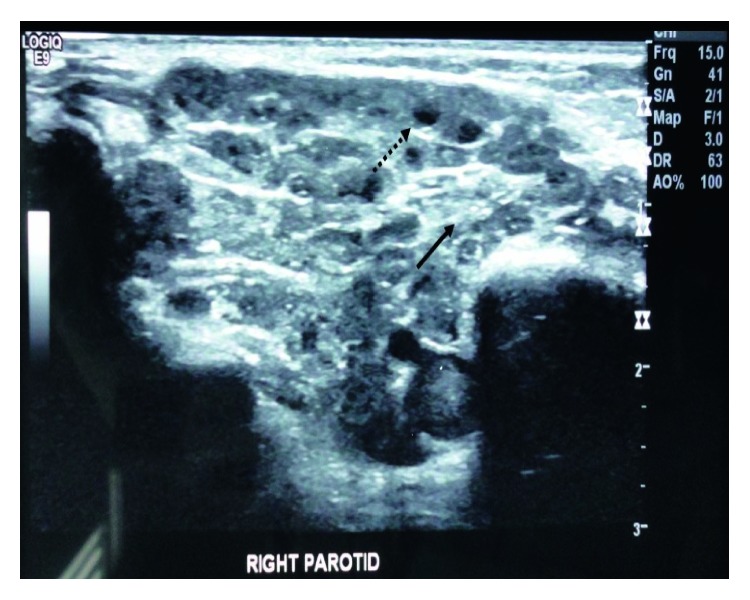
Ultrasonography image showing the parotid gland with low, heterogeneous echogenicity (
